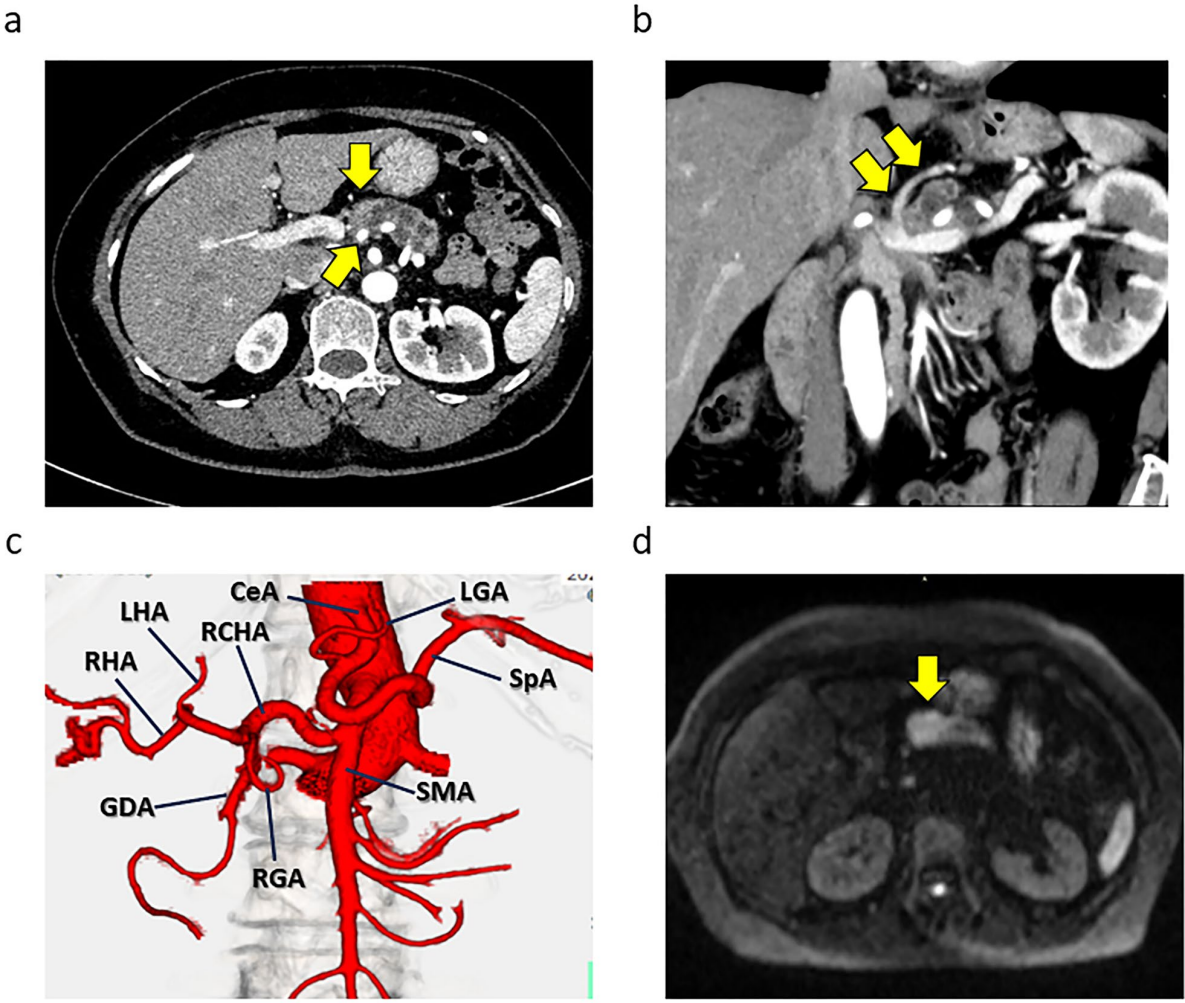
Computed tomography (CT) and magnetic resonance imaging (MRI) of the case. a The enhanced CT detected the pancreatic body tumor and main pancreatic duct dilation in the pancreatic body and tail. The arrows indicated that the tumor was in contact with splenic artery (SpA). b The enhanced CT shows that the tumor was in contact with the left gastric vein (LGV). The arrows indicated the LGV. c Three-dimensional artery image from enhanced CT. Celiac artery (CeA), left gastric artery (LGA), SpA, superior mesenteric artery (SMA), replaced common hepatic artery (RCHA), left hepatic artery (LHA), right hepatic artery (RHA), gastroduodenal artery (GDA) and right gastric artery (RGA) were indicated. d MRI diffusion-weighted image revealed high intensity in the pancreatic body. The arrow indicated the tumor
